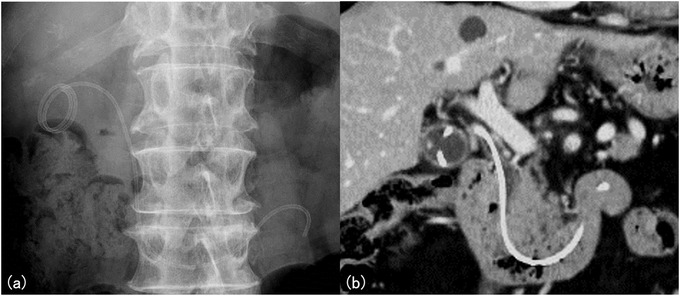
(a) A same case as observed in Figure 3. Plain radiography of the abdomen 3 months after the procedure. The distal end of the stent migrated to the third portion of the duodenum. (b) Computed tomography images revealed that the tip of the stent remained in the gallbladder. There was no cholecystitis relapse. We recommended stent replacement. However, the patient refused. Hence, follow‐up examination was performed
